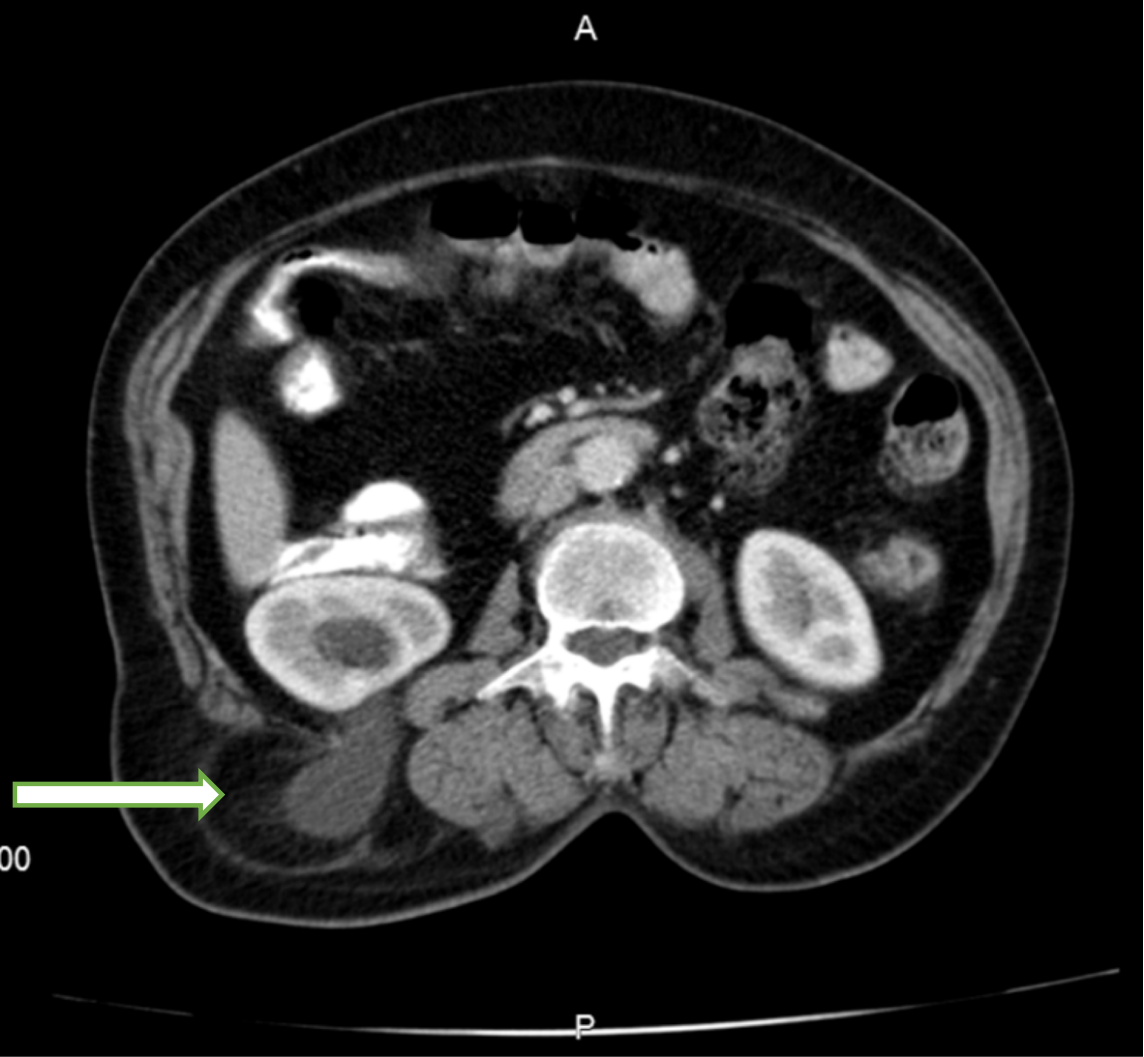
Spiral CT scan without contrast: herniated sac containing retroperitoneal and omental fat, and right renal pelvis (white arrow)
